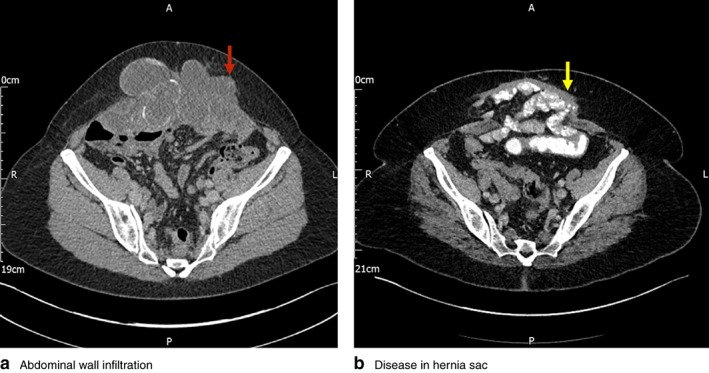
CT images demonstrating a abdominal wall infiltration by tumour (red arrow) and b incisional hernia with disease within hernia sac (yellow arrow)
